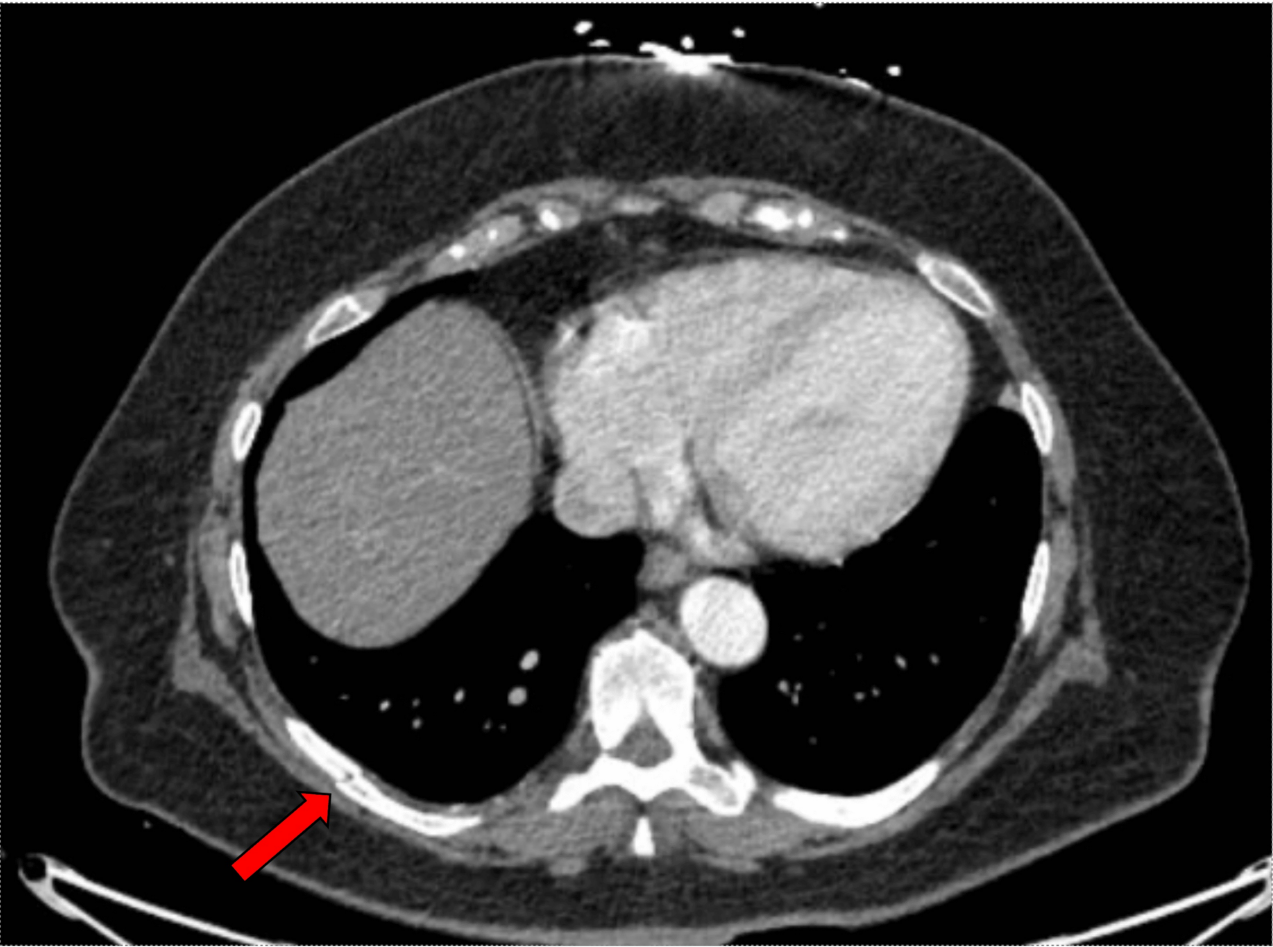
CTA of the chest on admission A CTA of the chest shows an acute fracture in the right ninth rib. CTA: Computed tomography angiography
